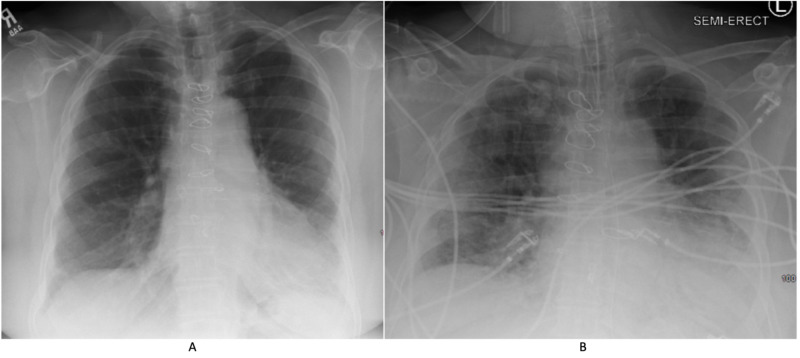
Chest X-ray (A) Chest X-ray done three months prior to presentation shows bilateral interstitial infiltrates predominantly in the lung bases. (B) Chest X-ray done on day 4 of COVID-19 shows worsening bilateral multifocal infiltrates superimposed on chronic changes.
